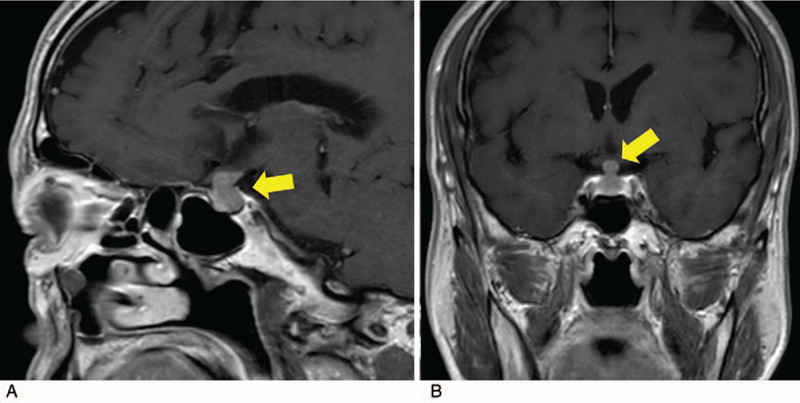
Gadolinium-enhanced T1-weighted brain magnetic resonance images obtained on admission (A: sagittal image, B: coronal image). The images reveal a thickened pituitary stalk and a hyperintense area in the enlarged pituitary gland (arrows).
